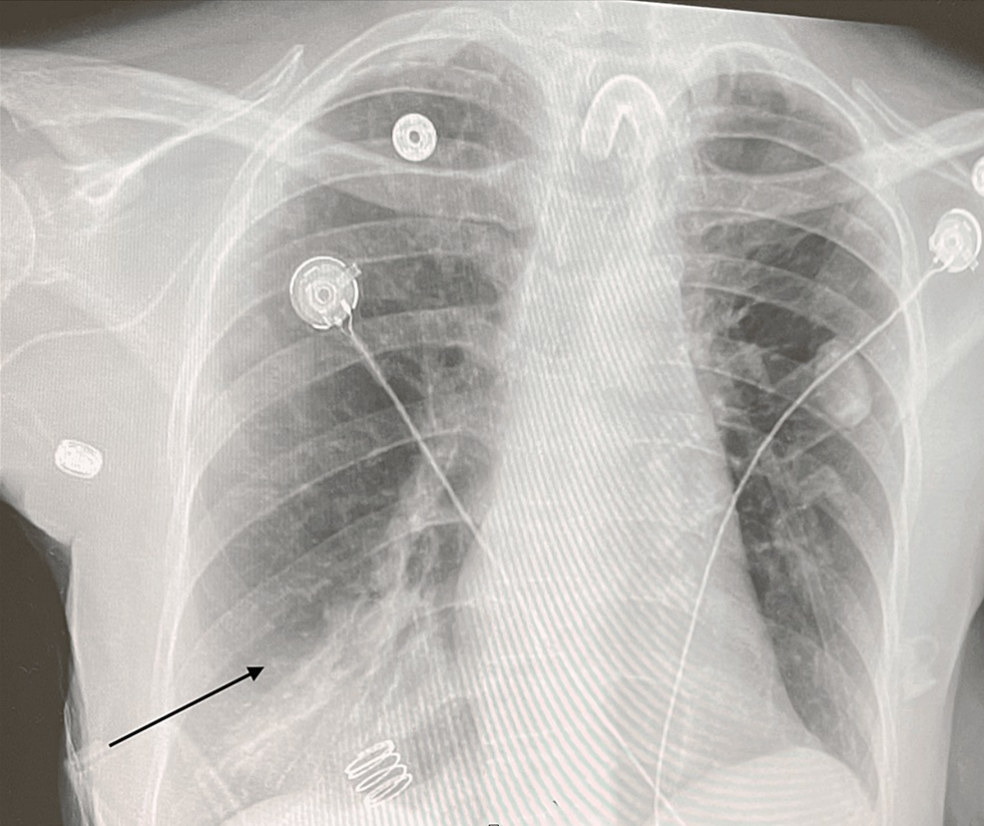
Chest X-ray of the patient presenting right lower lung pneumonia
